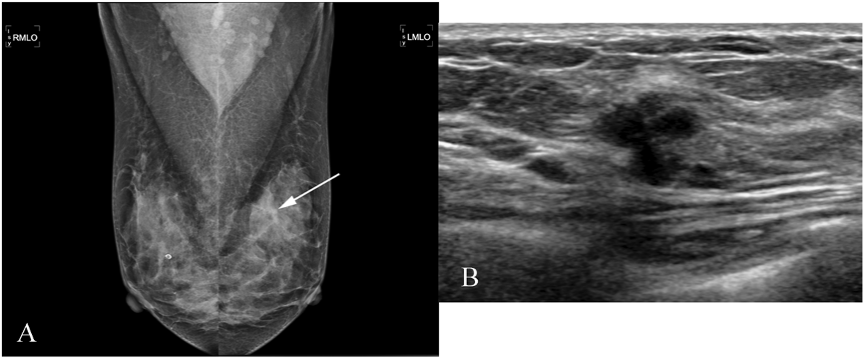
Radiologic findings of the left breast. (A) Mammography showing a focal asymmetry in left upper breast and benign calcifications in both breasts (B) Ultrasonography showing irregular shaped and microlobulated hypoechoic small mass in left upper breast.
